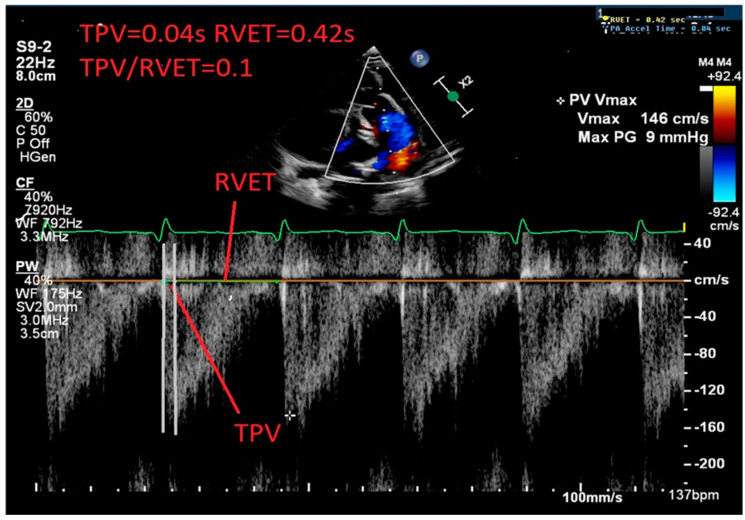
Pulmonary artery pulse Doppler trace showing a shortened time to peak velocity (TPV)/right ventricular ejection time ratio (RVET) TPV/RVET = 0.1 [TPV(0.04 s)/RVET(0.42 s) = 0.1, normal ≥ 0.31].
